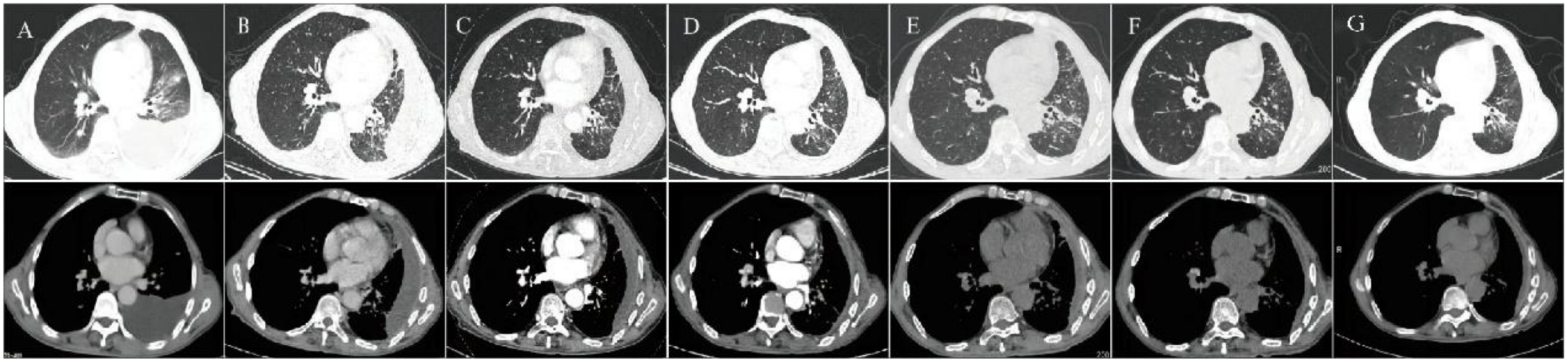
Serial chest CT scans of Case 2 during diagnosis and treatment. (A) Baseline chest CT prior to targeted therapy. (B) After 3 months of osimertinib (January 31, 2024). (C) After 4 cycles of furmonertinib plus pemetrexed (May 10, 2024). (D) Month 9 of furmonertinib treatment (October 9, 2024). (E) Month 12 of furmonertinib treatment (February 27, 2025). (F) Month 16 of furmonertinib treatment (June 17, 2025). (G) Month 21 of furmonertinib treatment (November 20, 2025).
